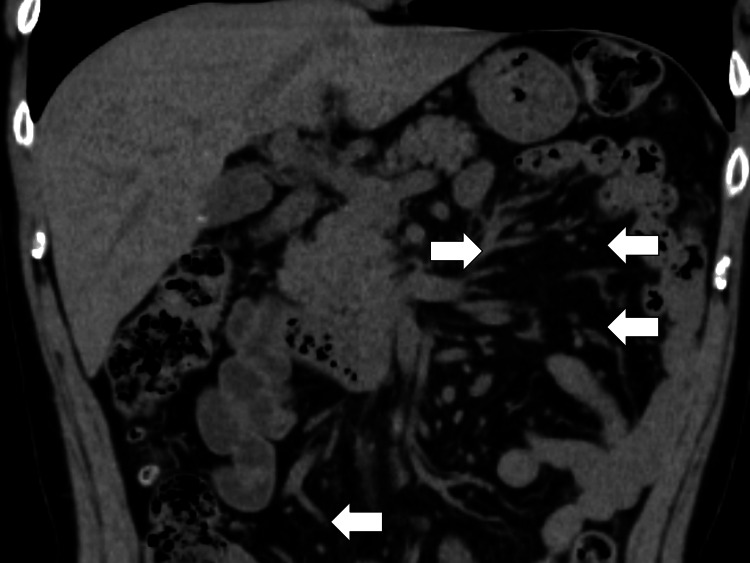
Follow-up abdominal computed tomography showing the decrease in swollen lymph nodes in the mesentery (white arrows)
